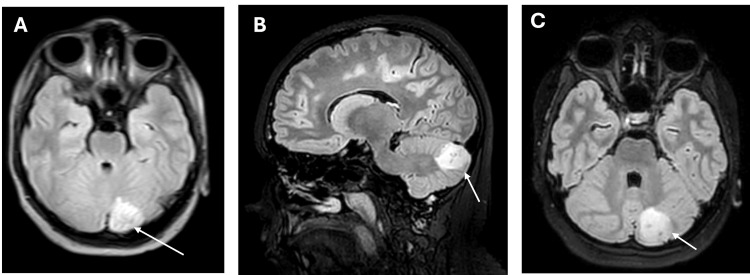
Follow-up brain MRI on day six demonstrating an evolving lesion in the left cerebellar hemisphere. (A) Axial FLAIR image on day six demonstrating interval evolution of the left cerebellar lesion with mild surrounding vasogenic oedema (white arrow). (B) Sagittal FLAIR image confirming the posterior fossa location with no fourth ventricular compression (white arrow). (C) Coronal FLAIR image demonstrating the extent of the evolving lesion (white arrow). No significant mass effect or hydrocephalus is present. FLAIR, fluid-attenuated inversion recovery
